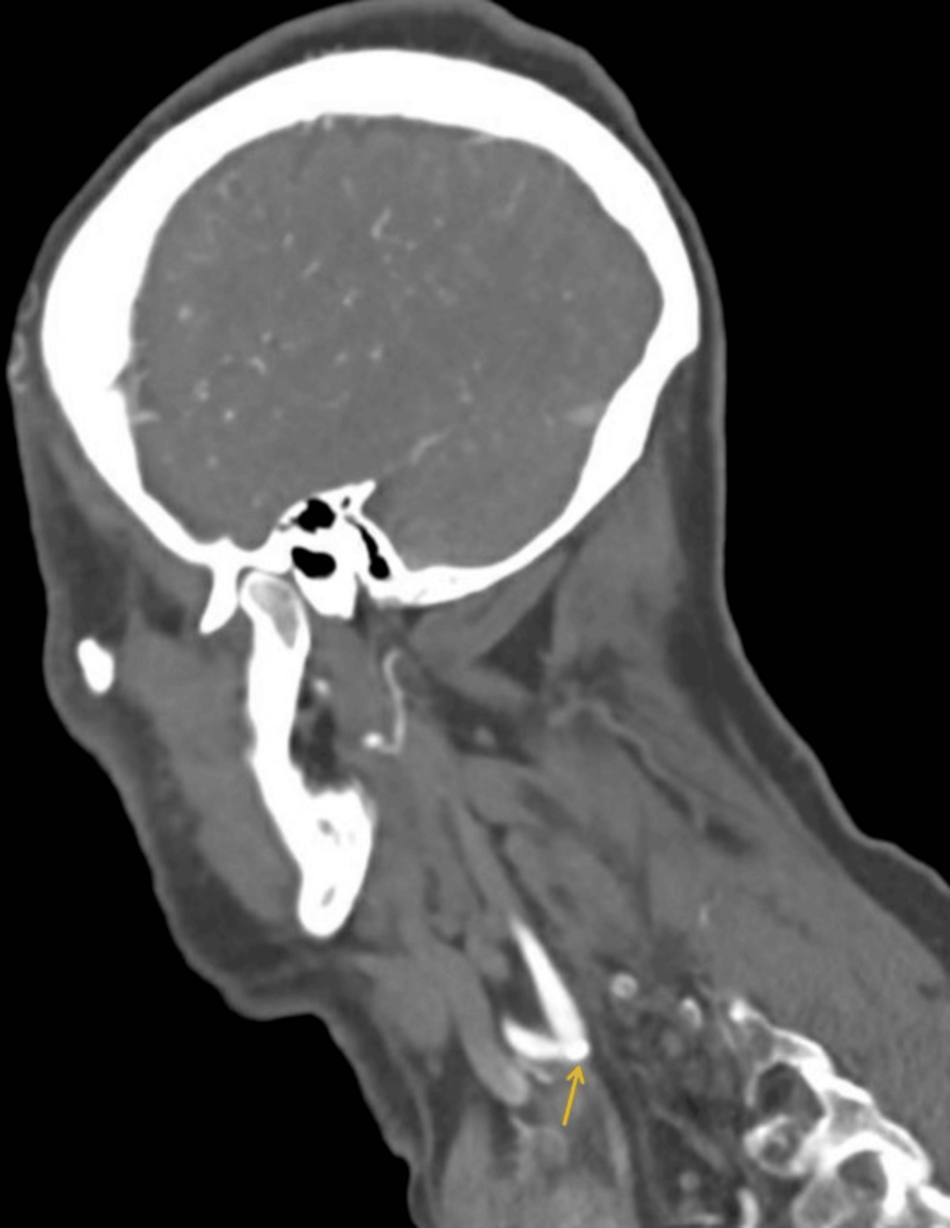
CT carotid angiogram sagittal section showing mild calcified atherosclerotic plaque just at the left carotid bifurcation. No significant intracranial stenosis or occlusion can be seen. CT: computed tomography
